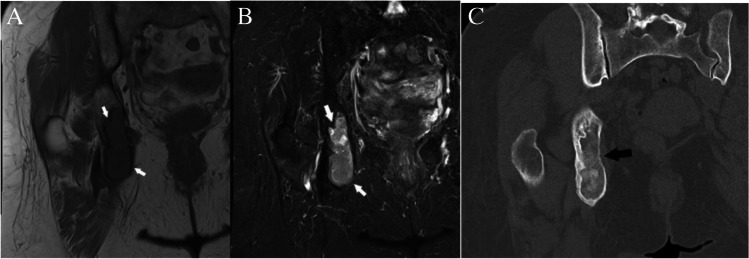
Case 5. Fibrous dysplasia. 84-year-old female with right hip pain and clinical suspicion for hip osteoarthritis who underwent right hip MRI and subsequent CT for incidentally discovered lesion (arrows) in the right ischium. The lesion is isointense to muscle on the A coronal T1-weighted MR image and hyperintense on the B coronal T2-weighted fat-suppressed MR image. The lesion has a sclerotic rim and ground glass appearance on the C coronal CT image
